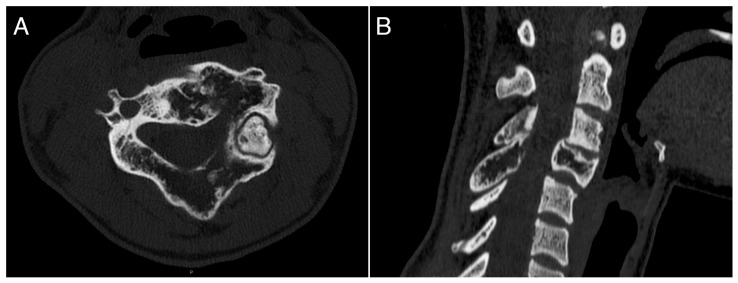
CT scan performed at seven months of treatment with denosumab, showing significant ossification of the lesion. (A): axial view; (B): sagittal view.
